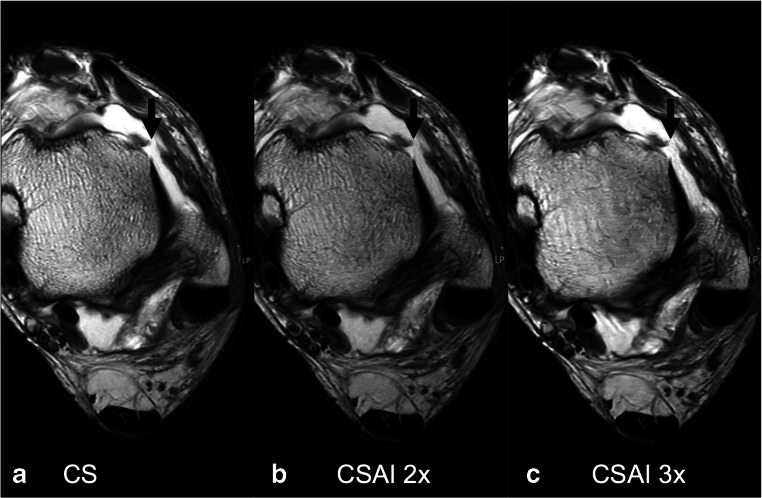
Axial T2-weighted TSE images of a 52-year-old male acquired using CS (a), CSAI2x (b), and CSAI3x (c), approximately reducing the acquisition time by half and two-thirds compared to CS, respectively. The images show a complete tear of the anterior talofibular ligament (black arrow). Note the slightly increased blurriness of the tibia on the CSAI3x images. However, the tear of the anterior talofibular ligament is clearly depicted on all three images
